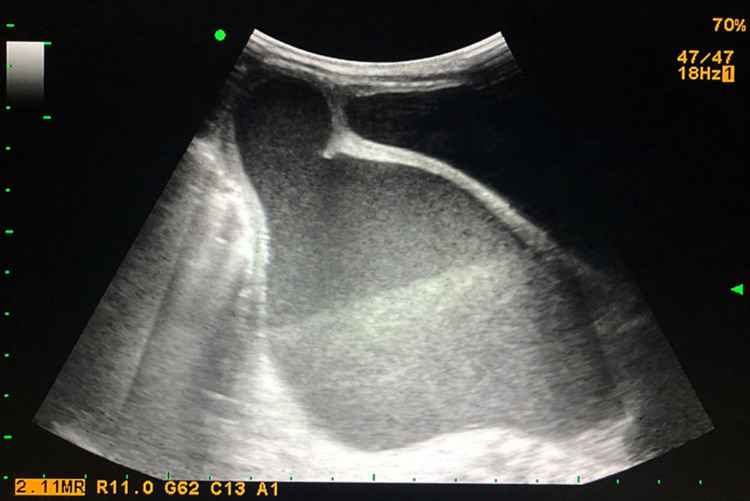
Patient's ultrasound with evidence of hydro-hematocolpos that extended into the vaginal canal
